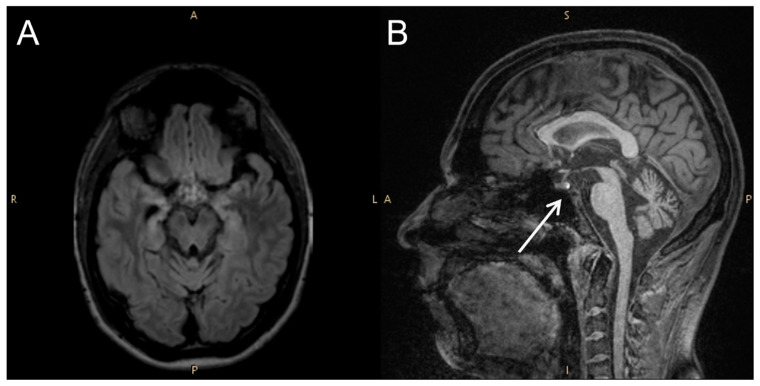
Follow-up brain MRI. Axial FLAIR image (A) showing bilateral atrophy of the hippocampus and amygdala. Sagittal T1 image (B) showing the normal hyperintense appearance of the neurohypophysis (arrow).
